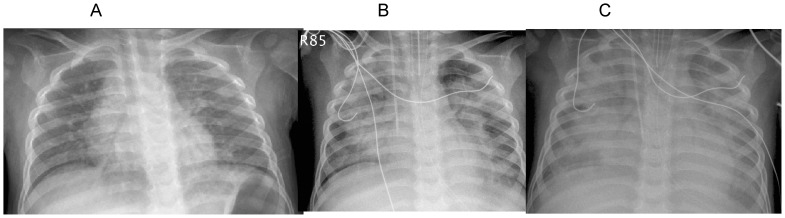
Serial chest radiographs of a 2 year-old boy: 2A shows bilaterally increased infiltration on Day 1 after onset, 2B reveals bilateral patch consolidation on Day 5, and 2C almost complete whiteout of bilateral lungs.
